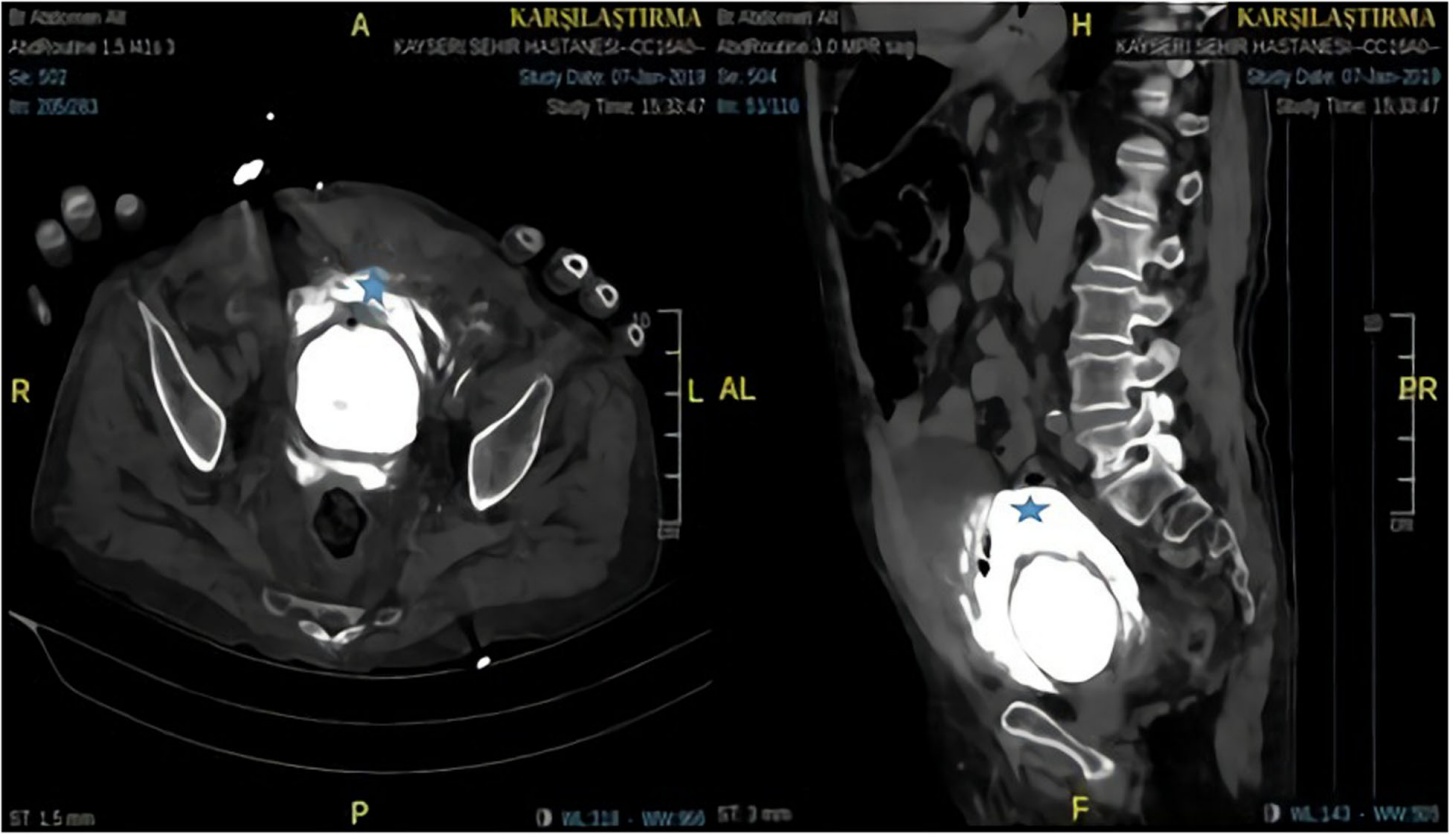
Abdominal CT with contrast images: axial and sagittal (*Contrast material leakage out of the bladder)
